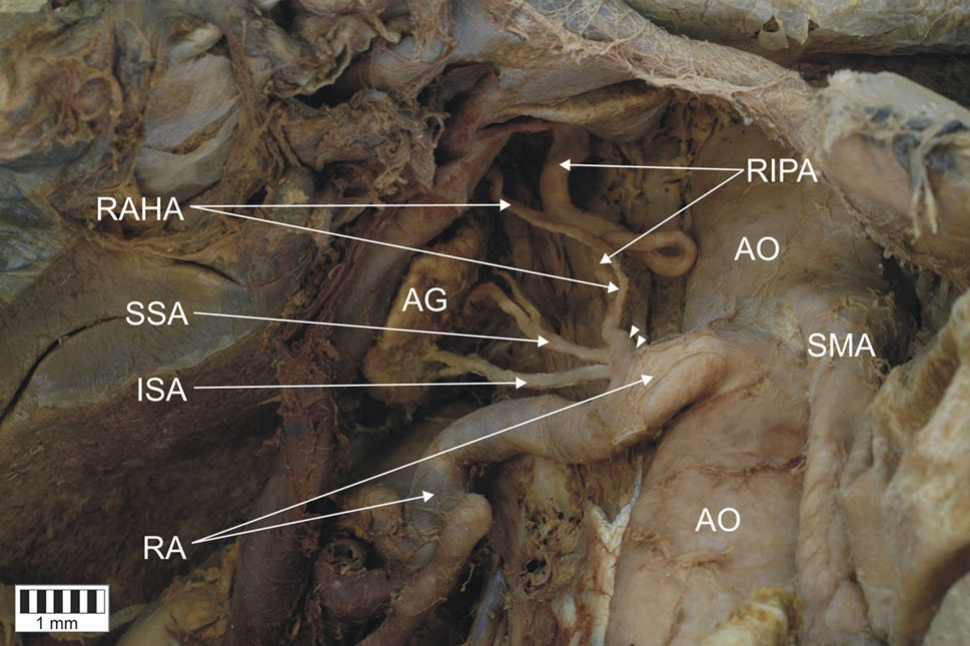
Origin of a common trunk for the right inferior phrenic artery (which gives rise to the right accessory hepatic artery) and inferior and superior suprarenal artery. White arrowheads present the common trunk for the right inferior phrenic artery, and inferior and superior suprarenal artery. *AO* abdominal aorta, *CT* celiac trunk, *SMA* superior mesenteric artery, *RIPA* right inferior phrenic artery, *RAHA* right accessory hepatic artery, *SSA* superior suprarenal artery, *ISA* inferior suprarenal artery, *RA* renal artery
